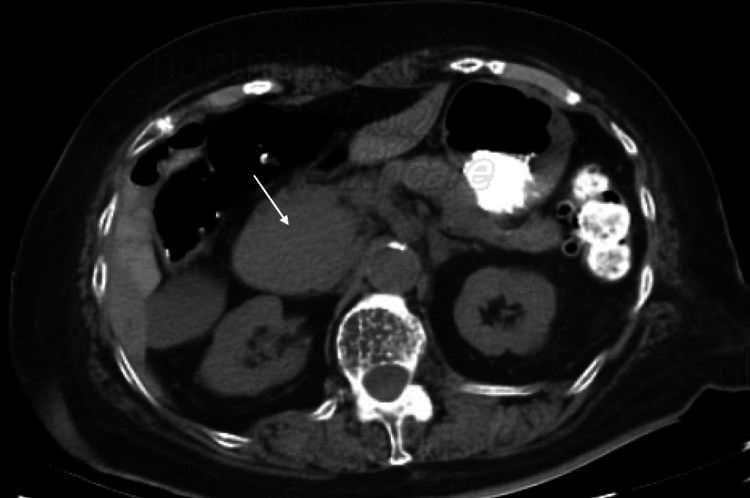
Axial computed tomography (CT) scan of the abdomen showing a portacaval mass (white arrow)
